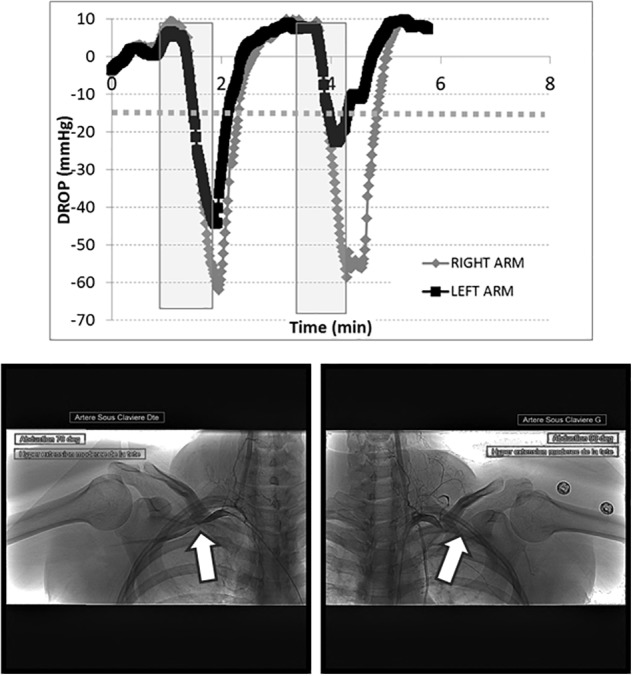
Transcutaneous oxygen pressure recordings are expressed as DROP values (mmHg) during two consecutive Roos maneuvers (gray squares). The *x*-axis represents the time in minutes. As shown, a dramatic decrease in the DROP values occurs during the Roos maneuvers on both sides, below –15 mmHg (dotted line). The corresponding arteriography shows the right (left panel) and left (right panel) sides, confirming occlusion of the subclavian artery during abduction of the arms (white arrows). This arteriography is from a 31-year-old woman who suffered for more than 4 years from upper limb pain, mostly on the right (dominant) side. She previously worked as a hairdresser, but had to stop this activity because of the pain. The pain originated at the shoulder and extended to the hands, with some paresthesias in the fourth and fifth digits. During the clinical examination, the Wright and Adson positions quickly led to a loss of the radial pulses. The Tinel test was negative. Bilateral TOS was suspected. A routine chest X-ray showed no abnormality of the cervico-thoracic junction. A duplex-scan demonstrated dynamic arterial and venous obstructions. Objective signs of lower plexus nerve lesions on electromyogram favored a neurogenic-type outlet syndrome. We also performed a dynamic bilateral angiography via a 4-Fr femoral puncture. This confirmed arterial outlet syndrome with an occlusive costoclavicular grip and a significant dynamic stenosis in the pectoralis minor muscle tunnel. She was prescribed an extended period of kinesiotherapy. DROP, decrease from the rest of oxygen pressure; TOS, thoracic outlet syndrome.
